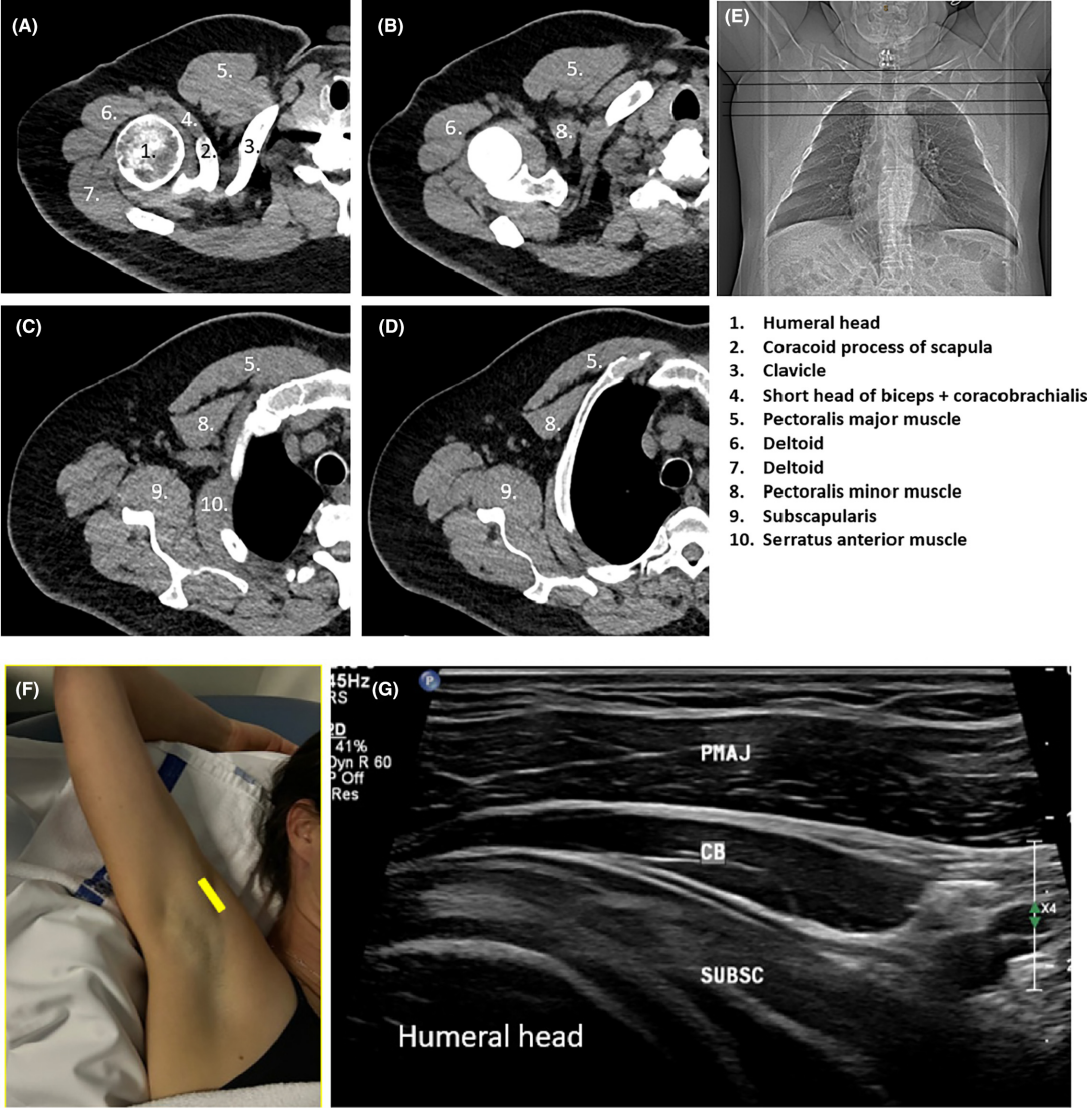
Imaging of the axilla. (A–D) Axial Computed Tomography (CT) images with the patient's arms positioned above their head (as shown in E). (F) Transducer position (yellow line) with arm above head. (G) Long axis sonographic image of the axilla obtained along the anterior axillary fold which demonstrates the coracobrachialis and short head of biceps muscle and tendon in long axis (should not be confused for the pectoralis minor muscle) overlying the humeral head. CB, coracobrachialis muscle; PMAJ, pectoralis major muscle; SUBSC, subscapularis muscle.
